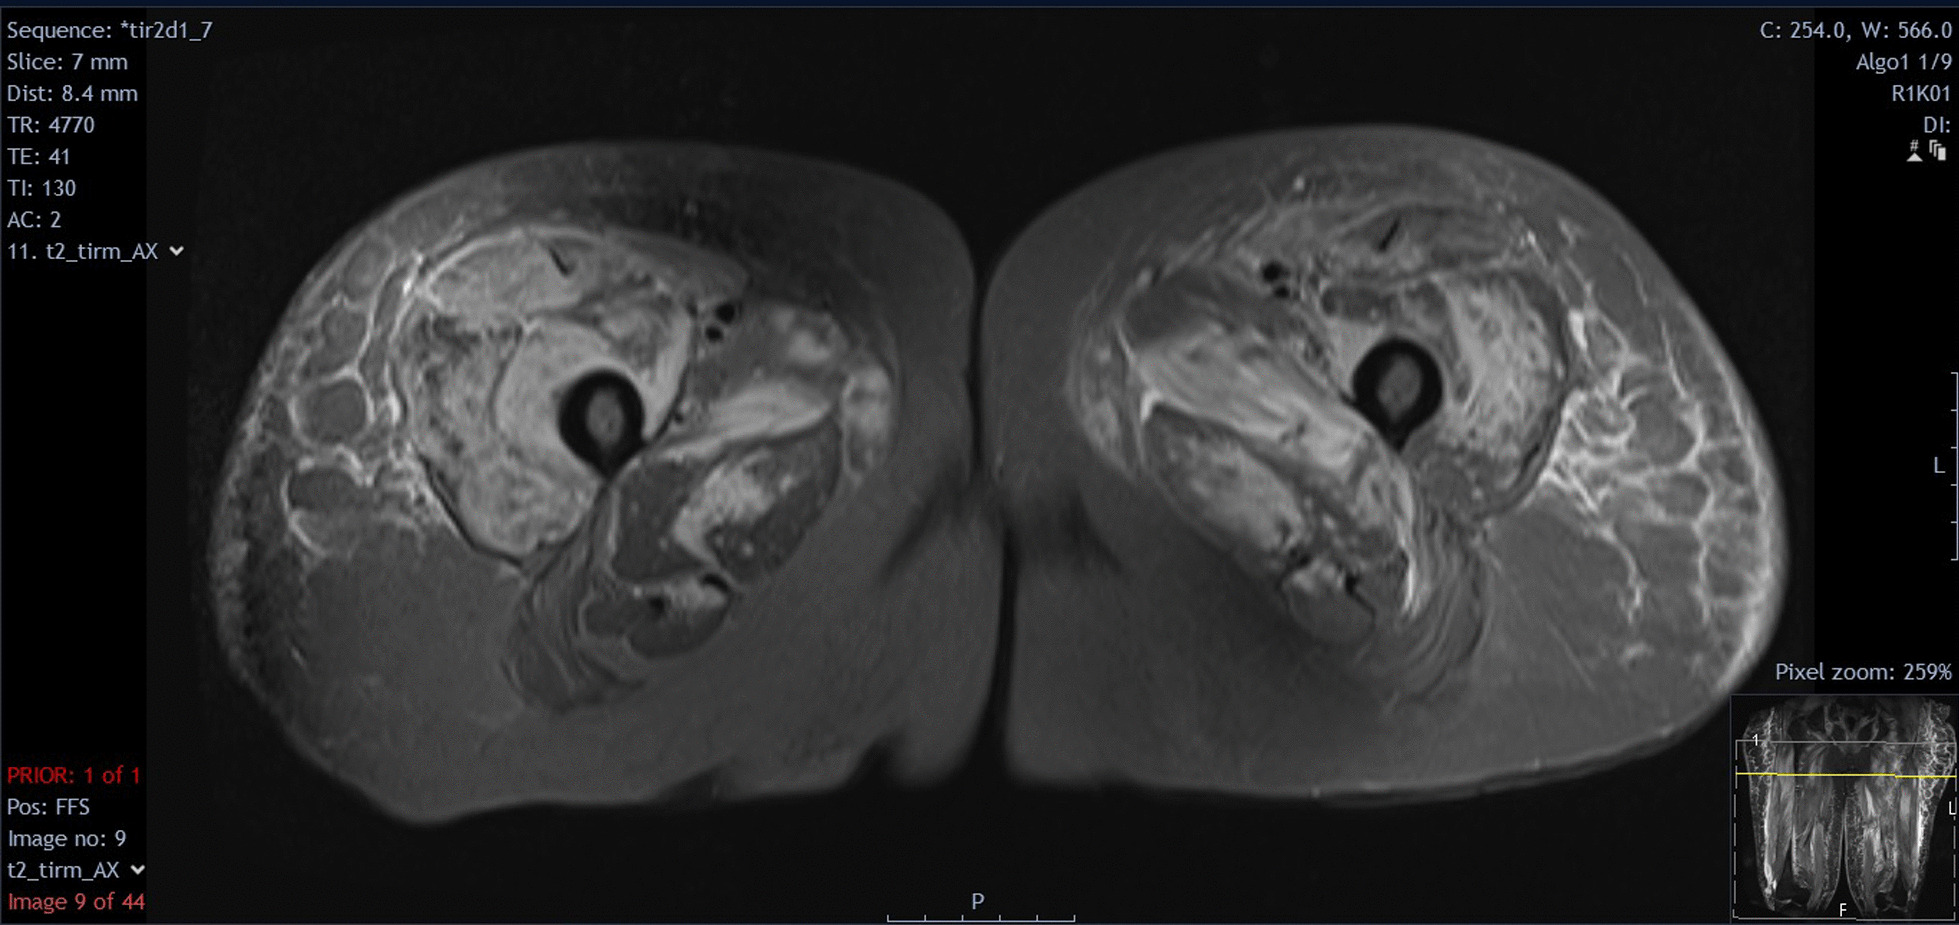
Magnetic resonance imaging T2 with Short Tau Inversion Recovery (STIR) sequences of patient’s proximal thighs showing edema in the vastus lateralis and gluteus muscles more pronounced on the right
